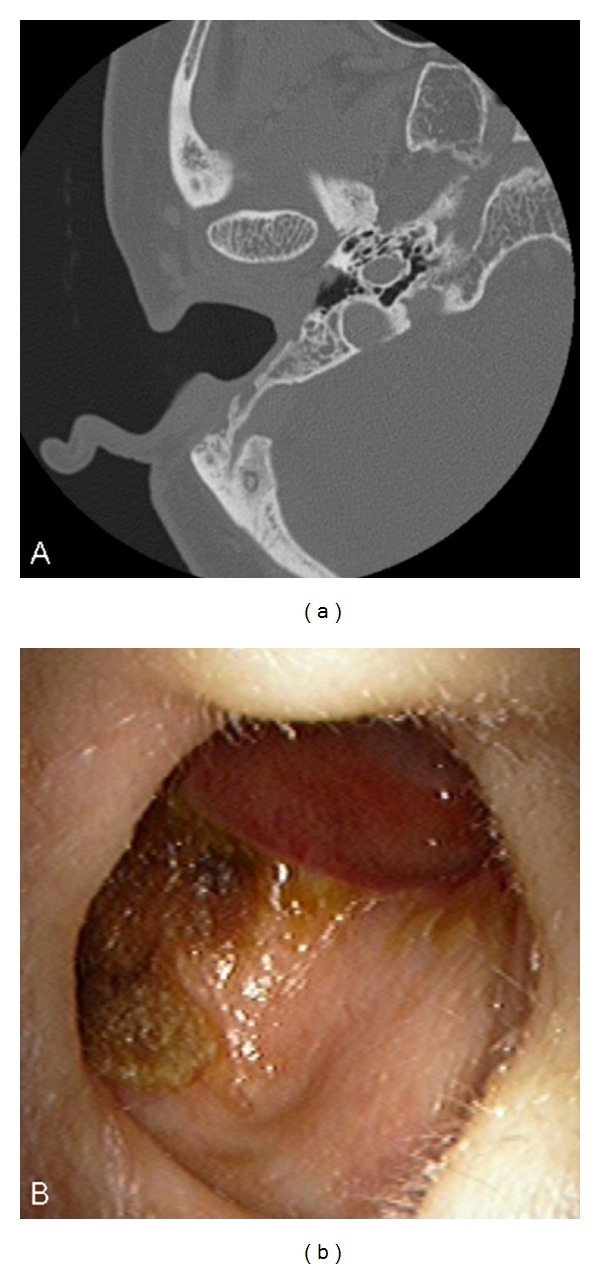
Six months after surgery in Case 1. (a) An axial CT image showed that the EAC had formed an open cavity with the mastoid bowl. (b) Although no stenosis or bone exposure was observed, a wet mucosal membrane and the crust were shown in the opened mastoid cavity.
